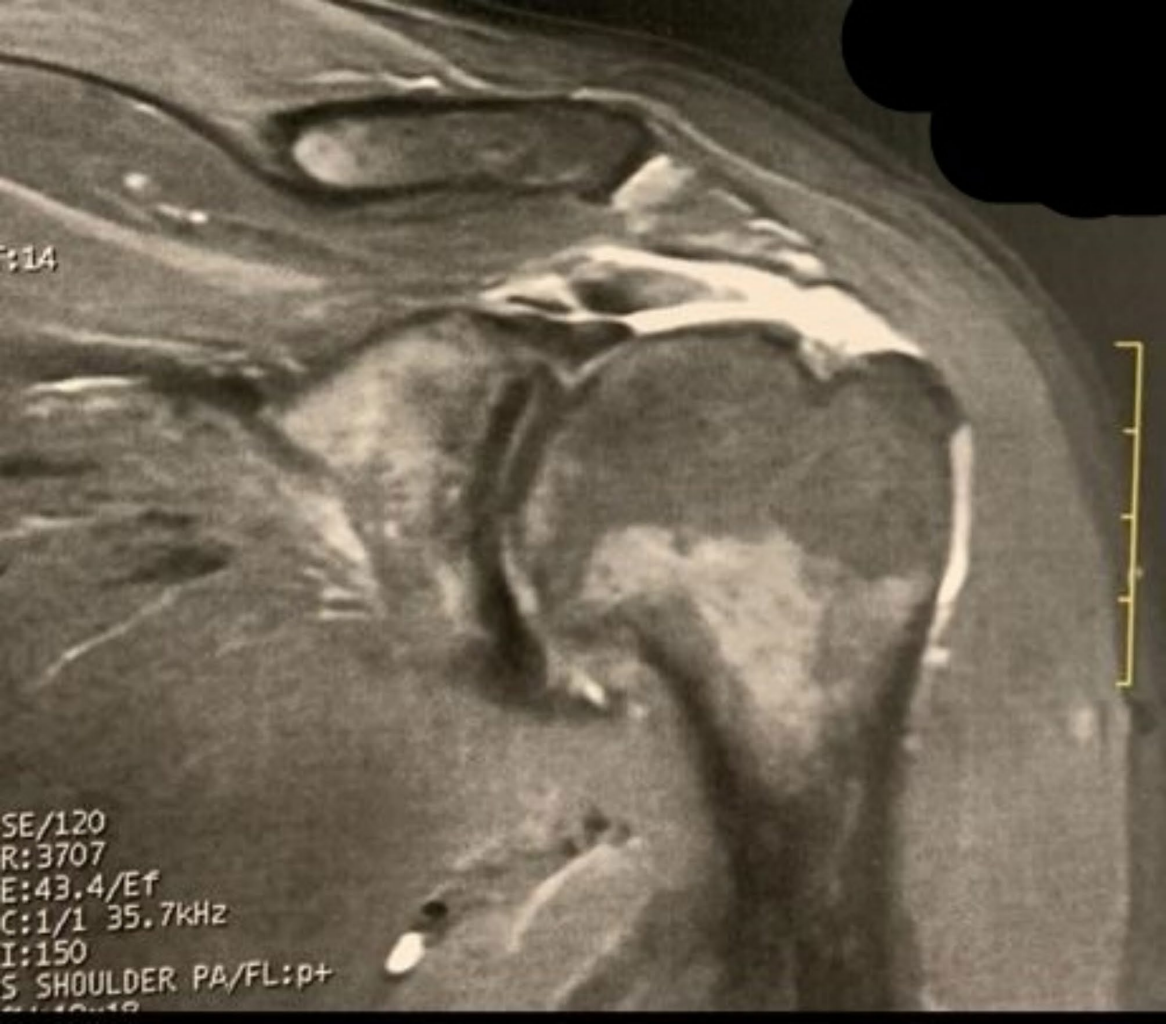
MRI coronal cut section showing a complete tear of the suprapinatus tendon.
